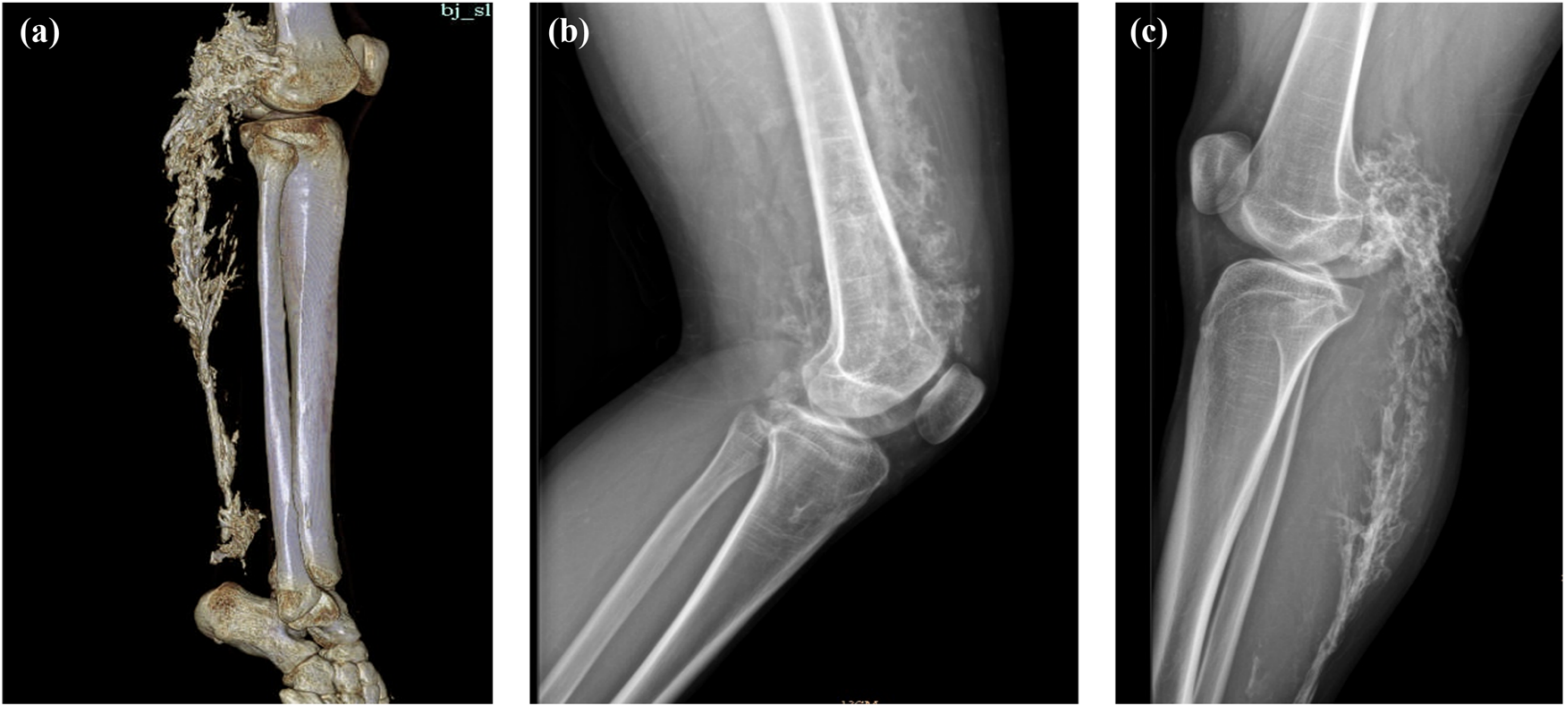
The three-dimensional reconstruction image (a) and the CT image (b and c) of the right knee joint of the patient showed multiple calcifications.
